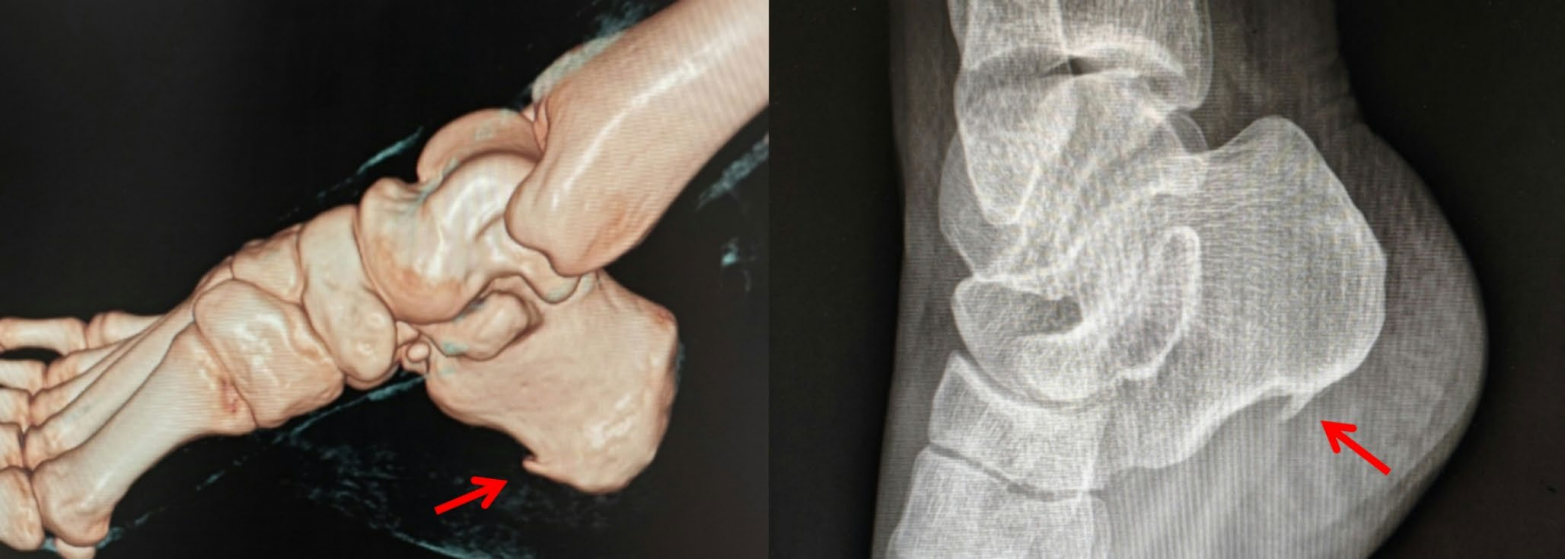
3D CT and X-ray images show calcaneal spurs (red arrow). Calcaneal spurs are bony outgrowths commonly associated with chronic stress or inflammation at the attachment sites of the plantar fascia or Achilles tendon
